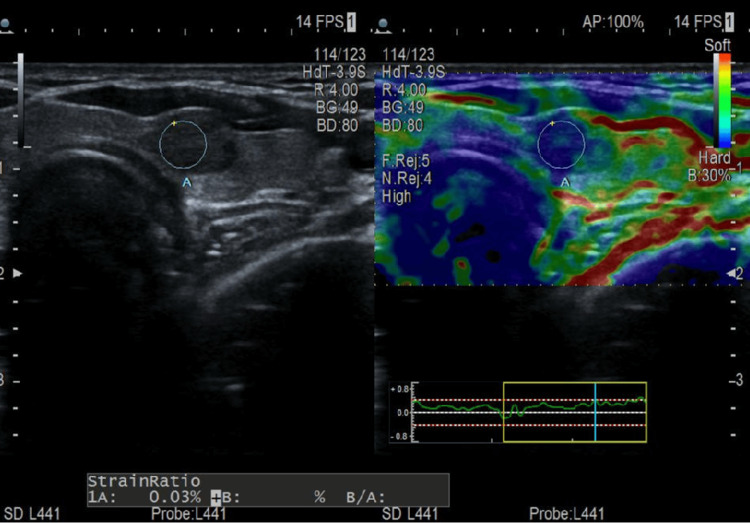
Thyroid ultrasound with elastography B-mode ultrasound with corresponding elastography image showing a suspicious left thyroid nodule with high stiffness (strain ratio: 0.03%), consistent with TIRADS 4c. TIRADS: Thyroid Imaging Reporting and Data System
